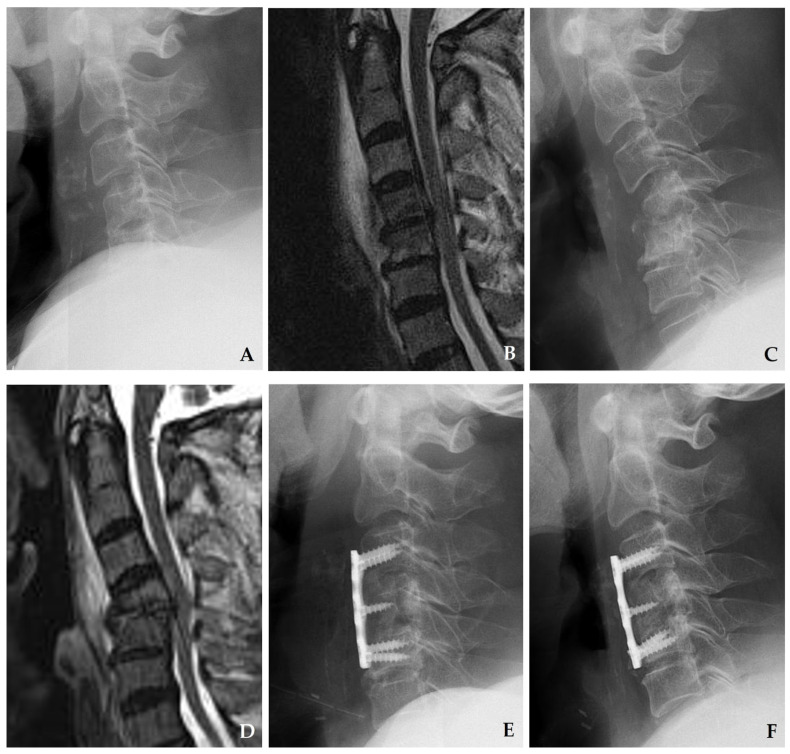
Initial plain radiograph (A) and magnetic resonance imaging (MRI) (B) demonstrate disc space narrowing at C4–C5 with a retropharyngeal abscess. After one month of antibiotic treatment, follow-up plain radiograph (C) and MRI (D) reveal progressive destructive changes in the C4–C5 vertebral bodies with kyphotic deformity, accompanied by retropharyngeal and epidural abscesses compressing the spinal cord. The patient underwent C3–C5 corpectomy with autograft and anterior plating (E), achieving solid fusion and complete resolution of spondylodiscitis at one year postoperatively (F).
